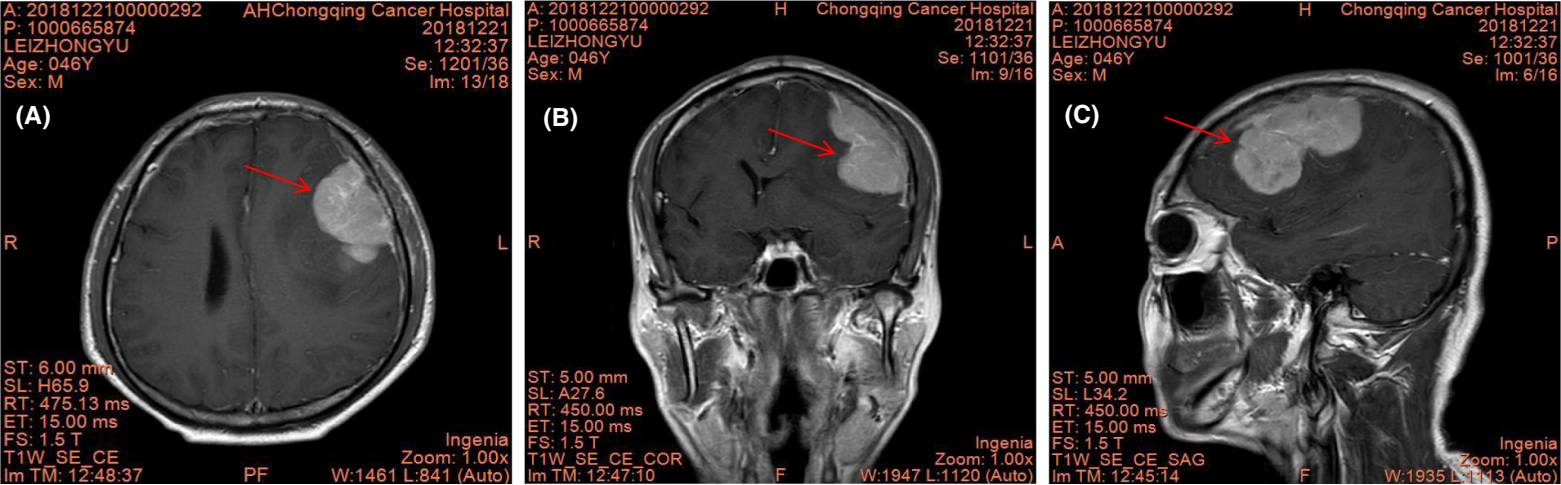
Magnetic resonance imaging show ill‐defined lesion in cranial meningeal and left frontal lobe. (A, Axial T1‐weighted axial; B, T1‑weighted coronal, C, T1‑weighted sagital planes; Red arrow means NCS involvement).
